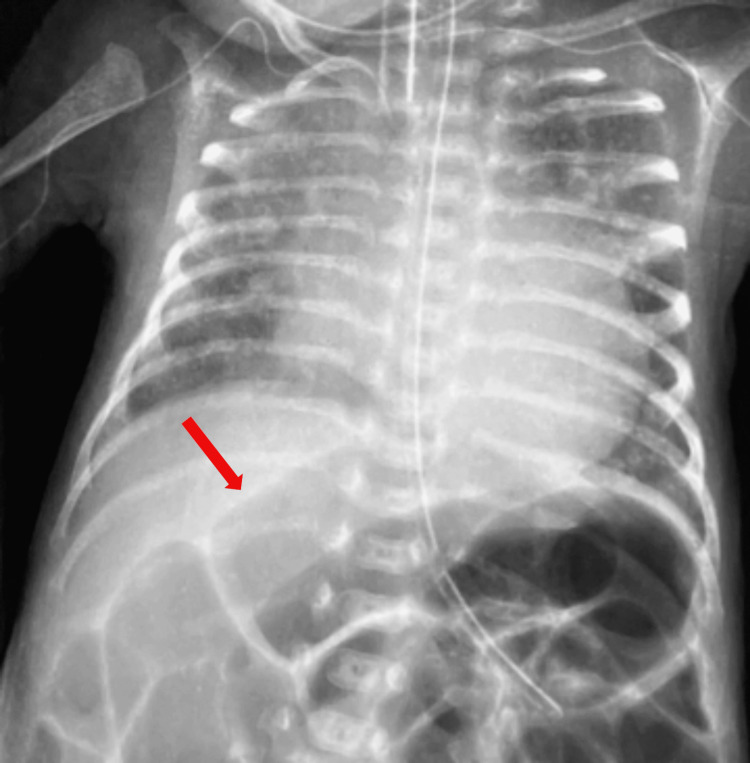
Initial chest radiography demonstrated marked cardiomegaly with a significant pulmonary plethora. Note the prominent congestive pattern across the lung fields and the presence of dilated, gas-filled bowel loops (red arrow).
